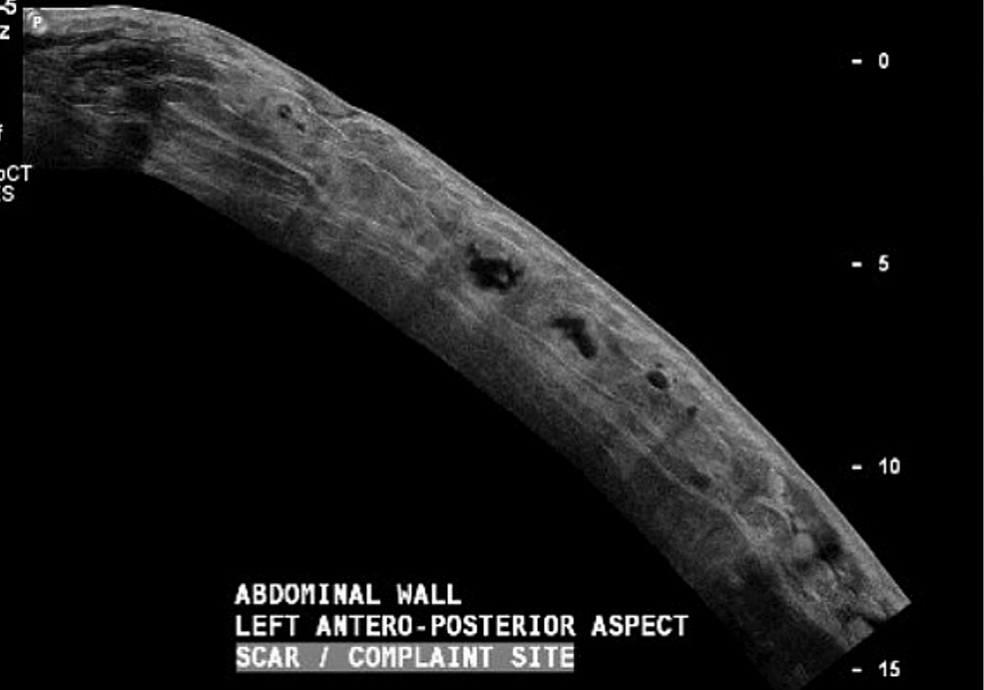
Another view of the ultrasound reflecting subcutaneous tissue involvement with gas accumulation
